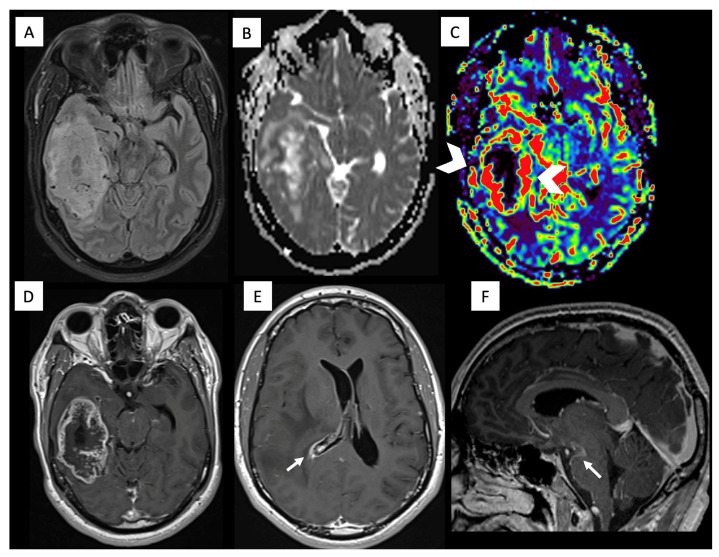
Brain MRI of Patient 3. Brain MRI axial FLAIR (A), ADC (B), DSC perfusion (C), and axial and sagittal contrast-enhanced T1-weighted (D–F) images show a temporal lobe mass with a necrotic center and peripheral enhancement, edema, and increased rCBV (arrowheads). Ependymal extension of the right ventricle and leptomeningeal extension are also noted (arrows).
